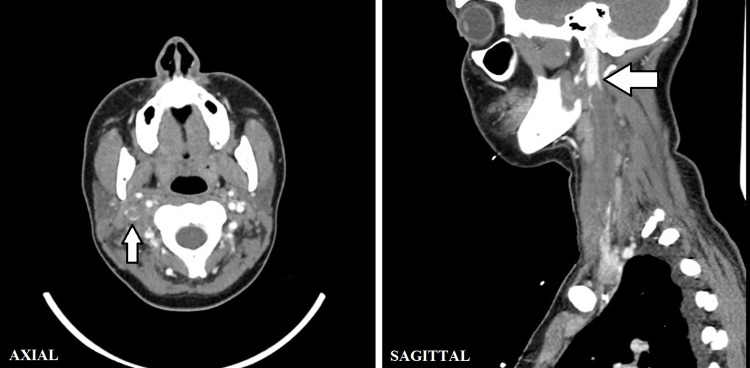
Axial and sagittal view of CT with contrast demonstrating a fully occluded right internal jugular vein (white arrows).
